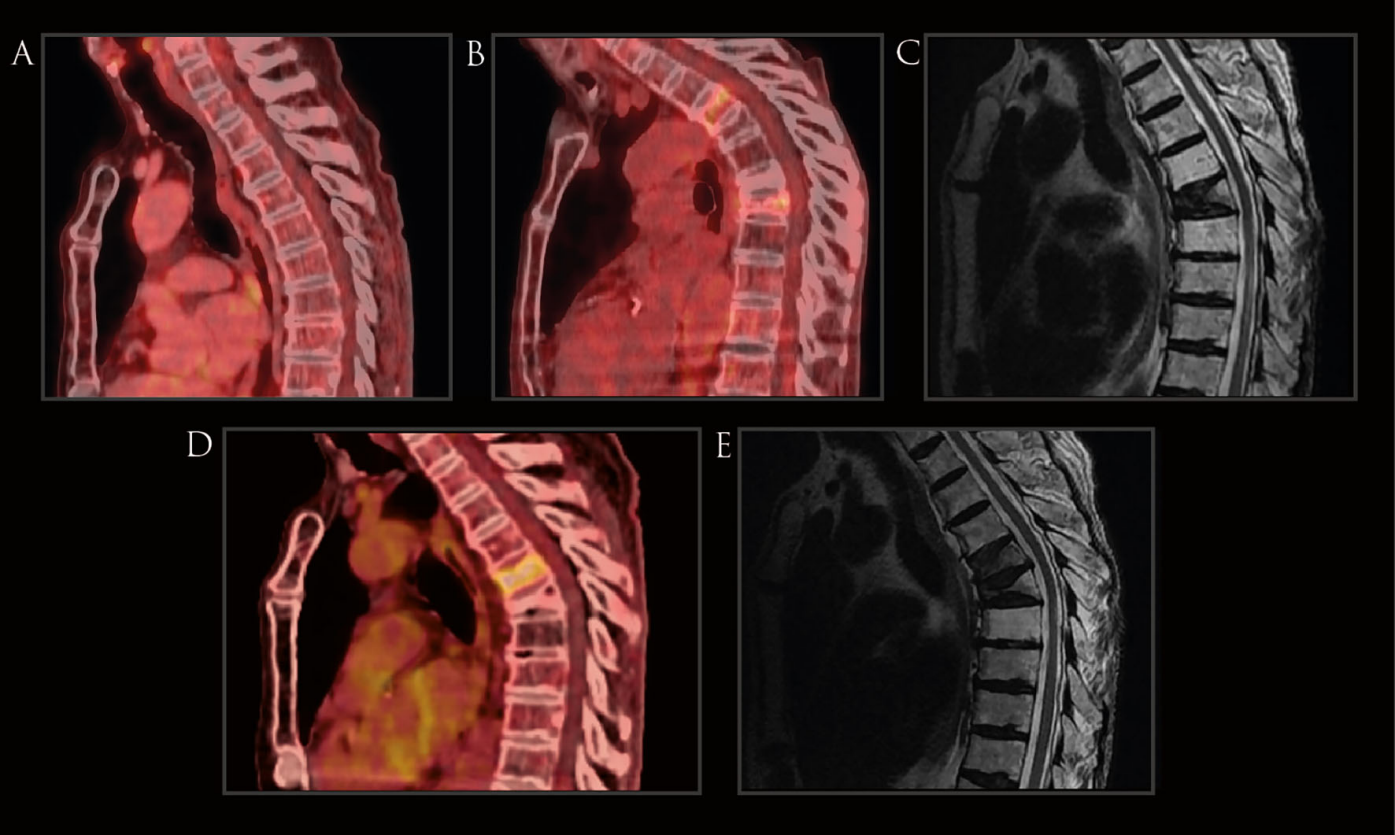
Imaging of Case 2. (A) PET-.CT scans conducted prior to radiotherapy revealed no evidence of vertebral fractures. (B) Follow-up PET-CT scans post-radiotherapy, depicting a compression fracture in the T7 vertebra, accompanied by diffuse metabolic activity, absent in prior imaging. (C) MRI showing the presence of the T7 vertebral fracture. (D) Follow-up PET-CT scan depicting new compression fracture in the T6 vertebra, accompanied by diffuse metabolic activity (E) MRI showing the presence of T6 and T7 compression fractures with anterior wedging.
